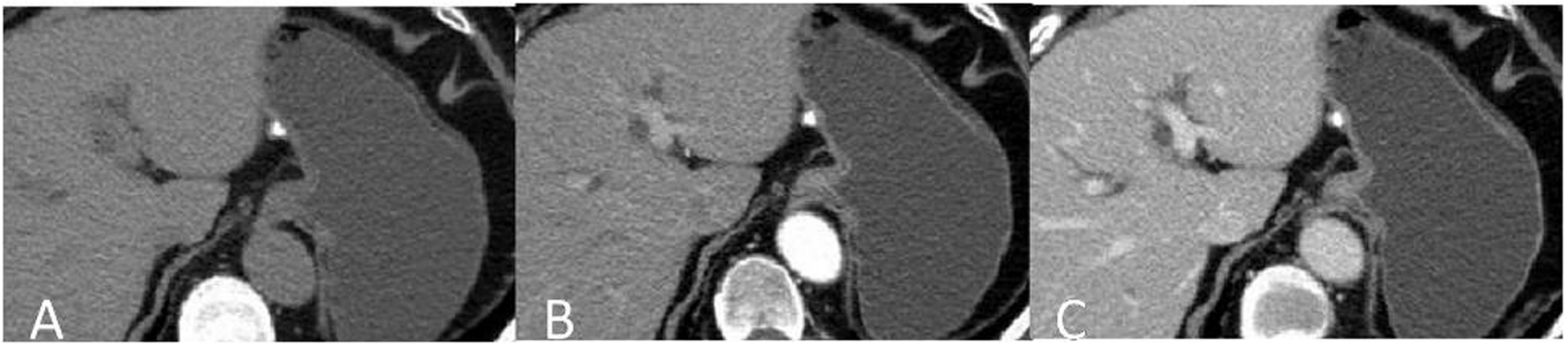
With a single stromal tumor under the serous membrane of the gastric fundus. A Unenhanced CT scan showed nodular calcifications in the gastric fundus subserosa protruding outside the stomach, with no apparent soft tissue around them. CT scan in the arterial phase (B) and portal vein phase (C) showed no heterogeneous enhancement around the lesion
